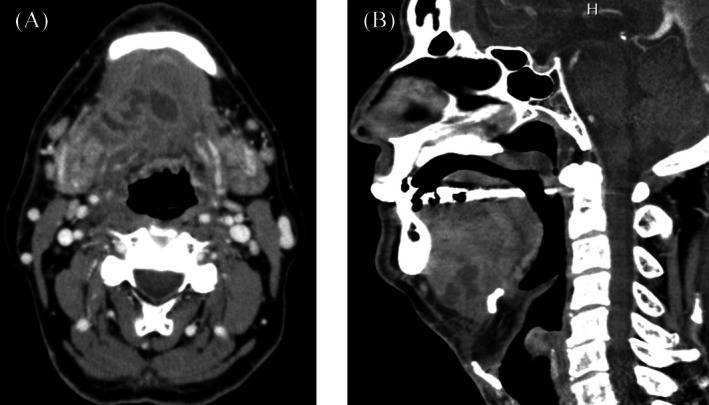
(A) Axial contrast‐enhanced computed tomography showing fluid collections in the floor of the mouth and submandibular spaces. (B) Sagittal contrast‐enhanced computed tomography demonstrating posterior displacement of the tongue due to soft tissue swelling in the submandibular and sublingual spaces.
